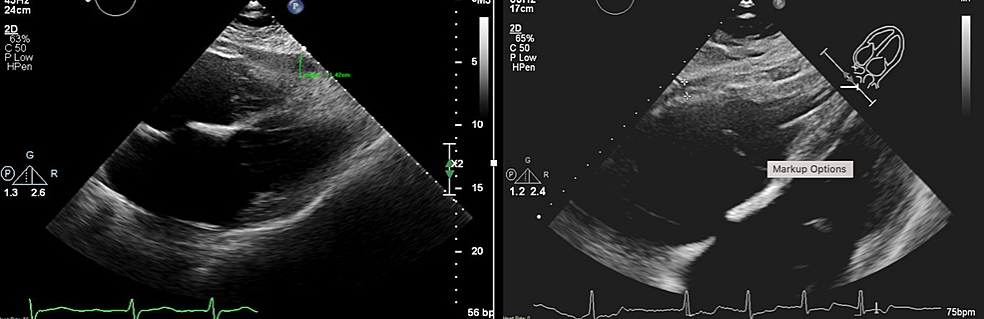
Left: Echocardiogram four months prior to procedure with chronic pericardial effusion. Right: Emergent post-procedure echocardiogram showing a new pleural effusion and a stable, small pericardial effusion without evidence of increased filling pressures.
